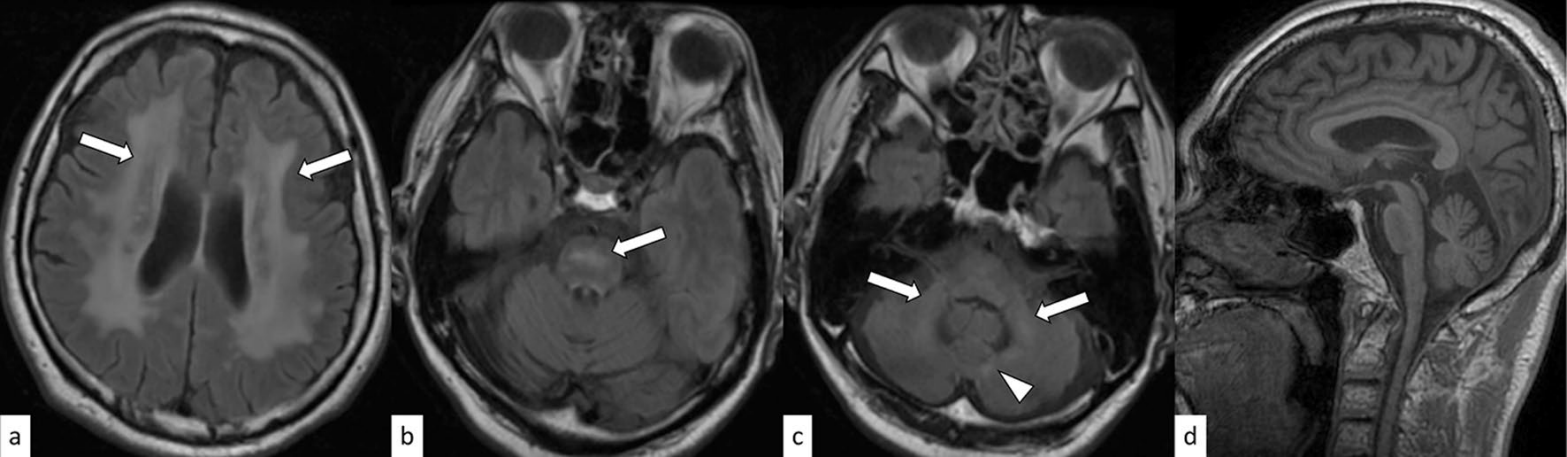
A 53-year-old man with DRPLA. FLAIR axial images show hyperintensity in the supratentorial white matter (a, arrows), pons (b, arrow), and cerebellar white matter (c, arrows). FLAIR hyperintensity in the paravermal area is observed (c, arrowhead). T1-weighted sagittal image shows atrophic brainstem (d)
